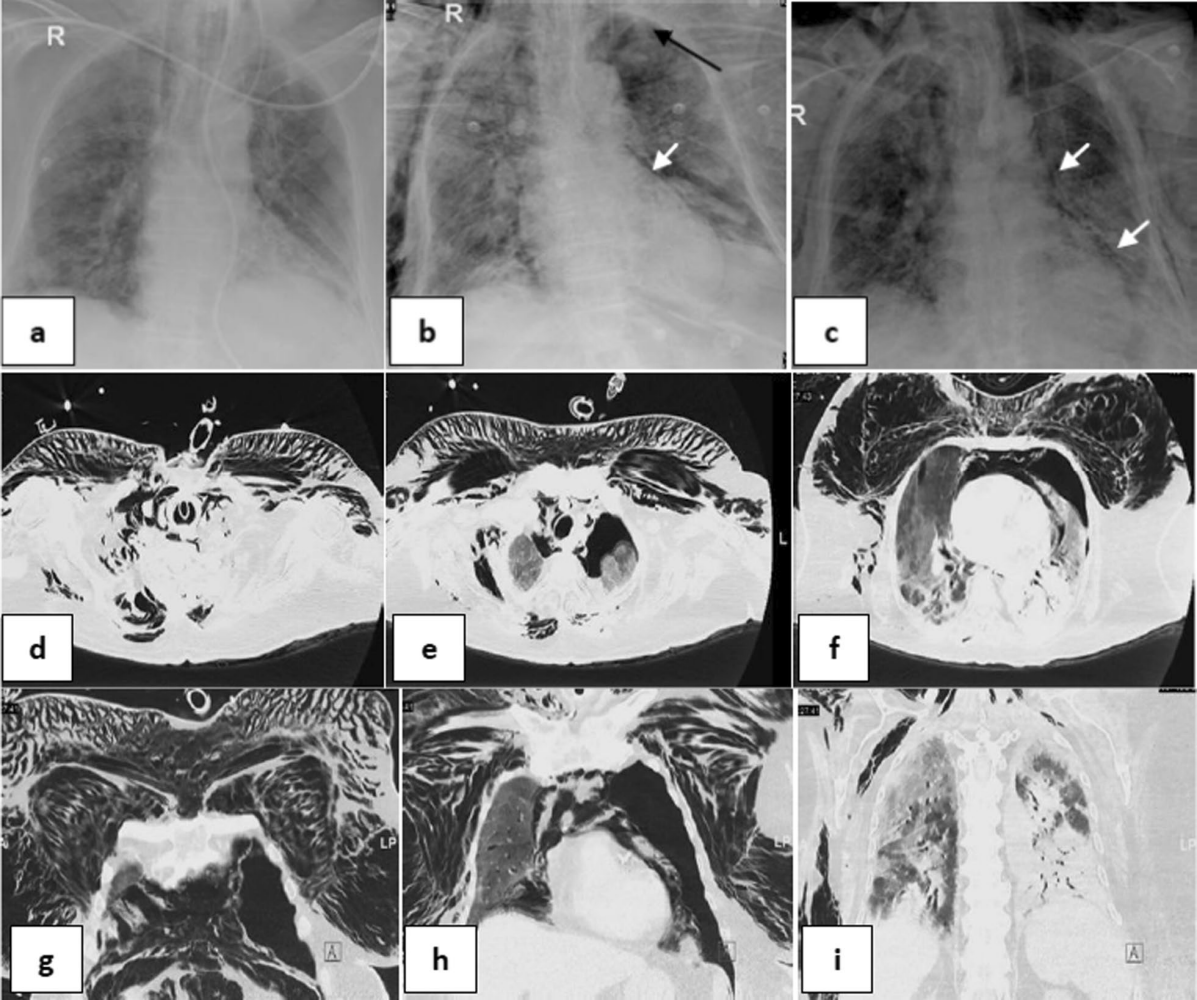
61-year-old male with hypertension and diabetes, intubated 5 days post admission. a–c serial frontal chest X-ray films demonstrate a left pneumothorax (black arrow), pneumomediastinum (white arrows) and surgical emphysema 8 days after intubation then progression of surgical emphysema. Note bilateral ill-defined air space opacities throughout both lungs. d–f & g–i axial and coronal reconstructing CT serial images, respectively, confirming the left pneumothorax, pneumomediastinum and extensive surgical emphysema
